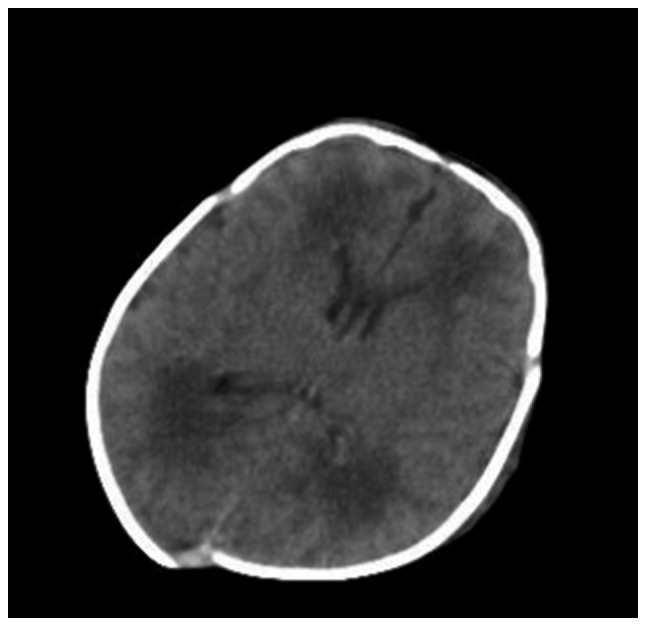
Standard dose computed tomography (120 kV, 250 mAsec).
